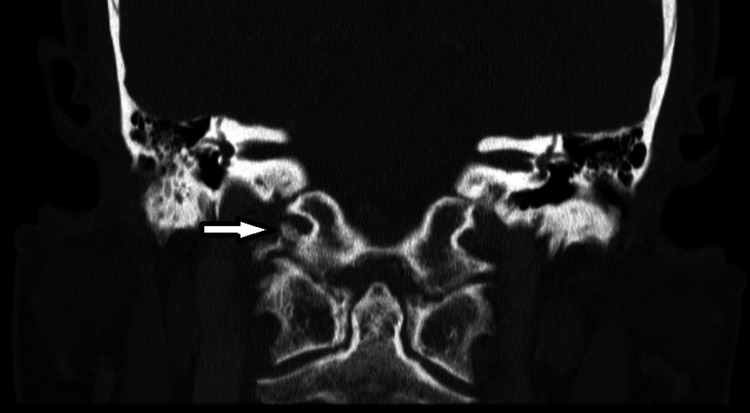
CT coronal section of the head and neck revealing AO osteophyte extending into the right jugular foramen with extrinsic compression and mild stenosis of the right internal carotid artery and jugular bulb. CT: computed tomography; AO: atlantooccipital
